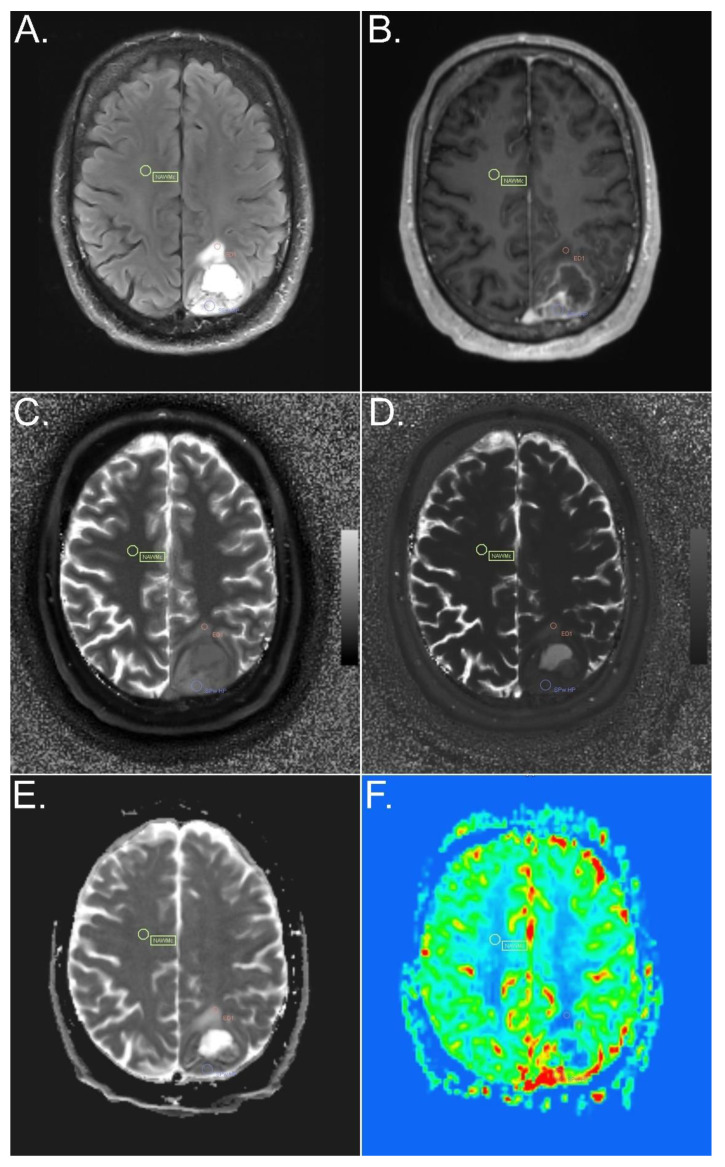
Example of ROI placement in different tumor components of a glioblastoma, IDH-wildtype. FLAIR (A), T1-weighted MPR post i.v. contrast administration (B), MRF T1 (C) and T2 (D) maps, ADC map (E), and perfusion-weighted imaging (F). ROIs were placed in the solid tumor part, in an area with contrast enhancement and an rCBV increase (violet circle), in peritumoral edema (red circle), and in contralateral NAWM (yellow circle). SPw HP, solid part of the tumor with contrast enhancement and with hyperperfusion; ED1, peritumoral edema less than or equal to 1 cm distant from the tumor; NAWMc, contralateral NAWM of the frontal lobe.
